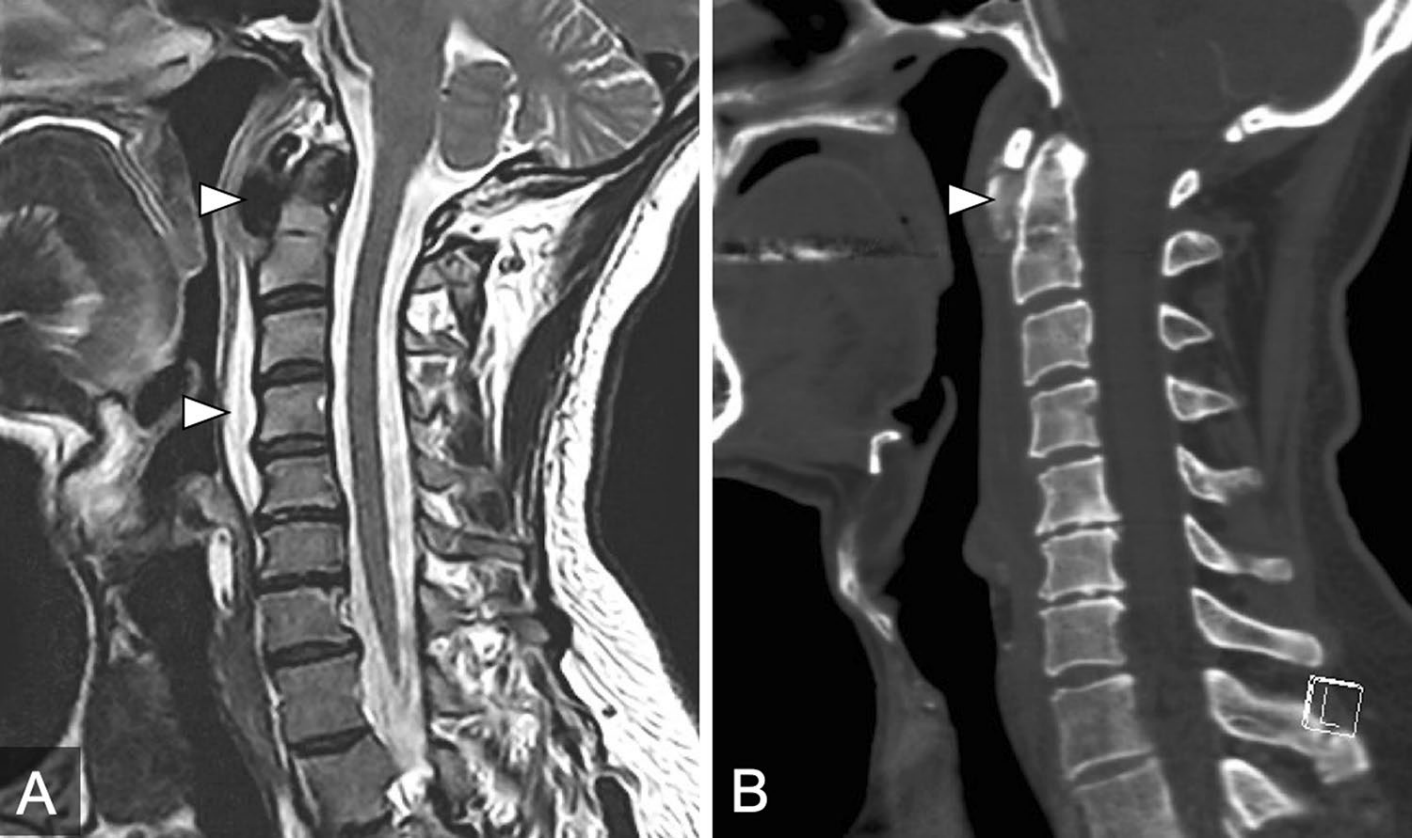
MRI and CT of a 56-year-old male patient with acute neck pain and impairment of neck movement (Patient 1). a Sagittal T2-weigthed image demonstrating prevertebral calcification at the level of C1/C2 (upper white arrowhead) with adjacent long sectional edematous prevertebral infiltration from C2–C5 (lower white arrowhead). Due to the diagnosis of a RA on the initial MRI, which was performed on an outpatient basis, he received intravenous antibiotic and analgetic therapy (Table 1). b Sagittal reconstruction of a CT scan in bone window setting demonstrating coarse prevertebral calcification at the level C1/C2 (white arrowhead). This follow-up CT was performed one day after treatment initiation. After the initiation of antibiotic and analgetic treatment, symptoms resolved within one week
